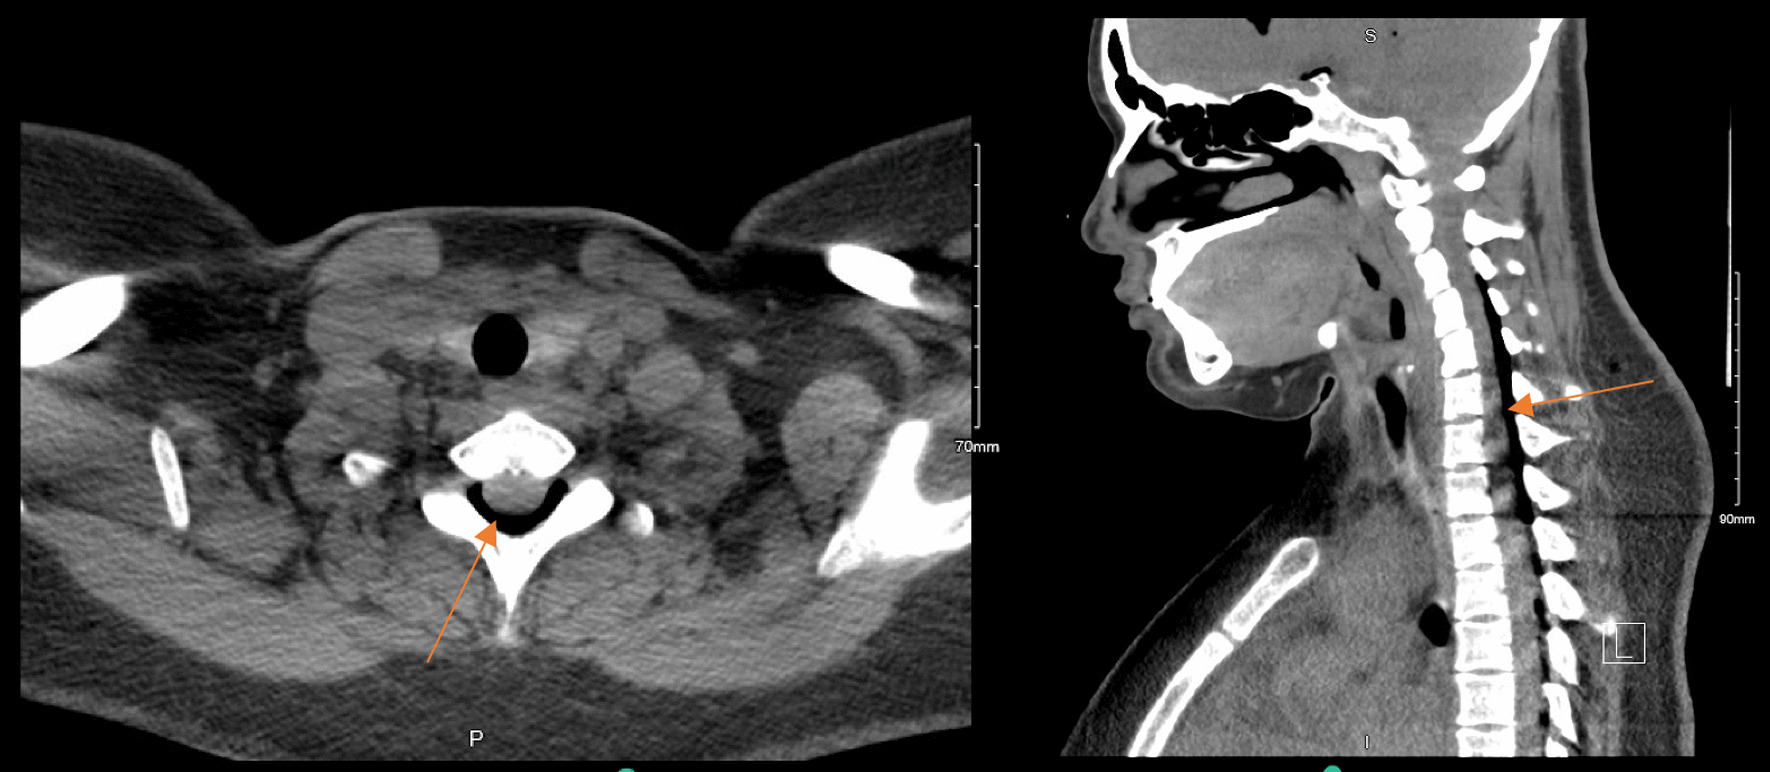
Computed tomography neck showing air within the cervical spinal canal. A Axial view, B Sagittal view. Orange arrows identify the abnormal presence of air
